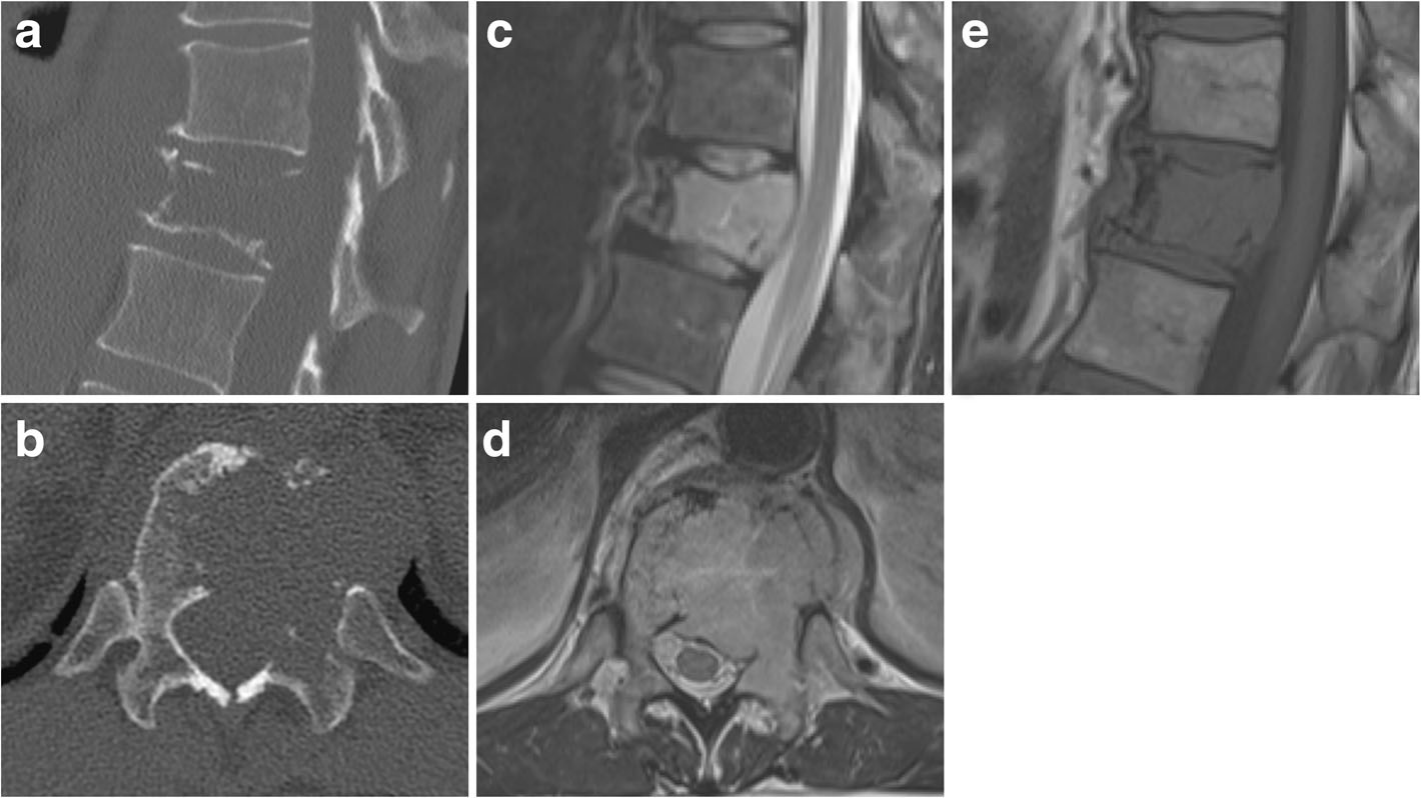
CT scan (a, b) of the lytic lesion of the T12 vertebra with unilateral posterolateral involvement. MRI with T2-hyperintense and T1-hypointense lesion (c, e) and more obvious involvement of the posterior elements (d)
